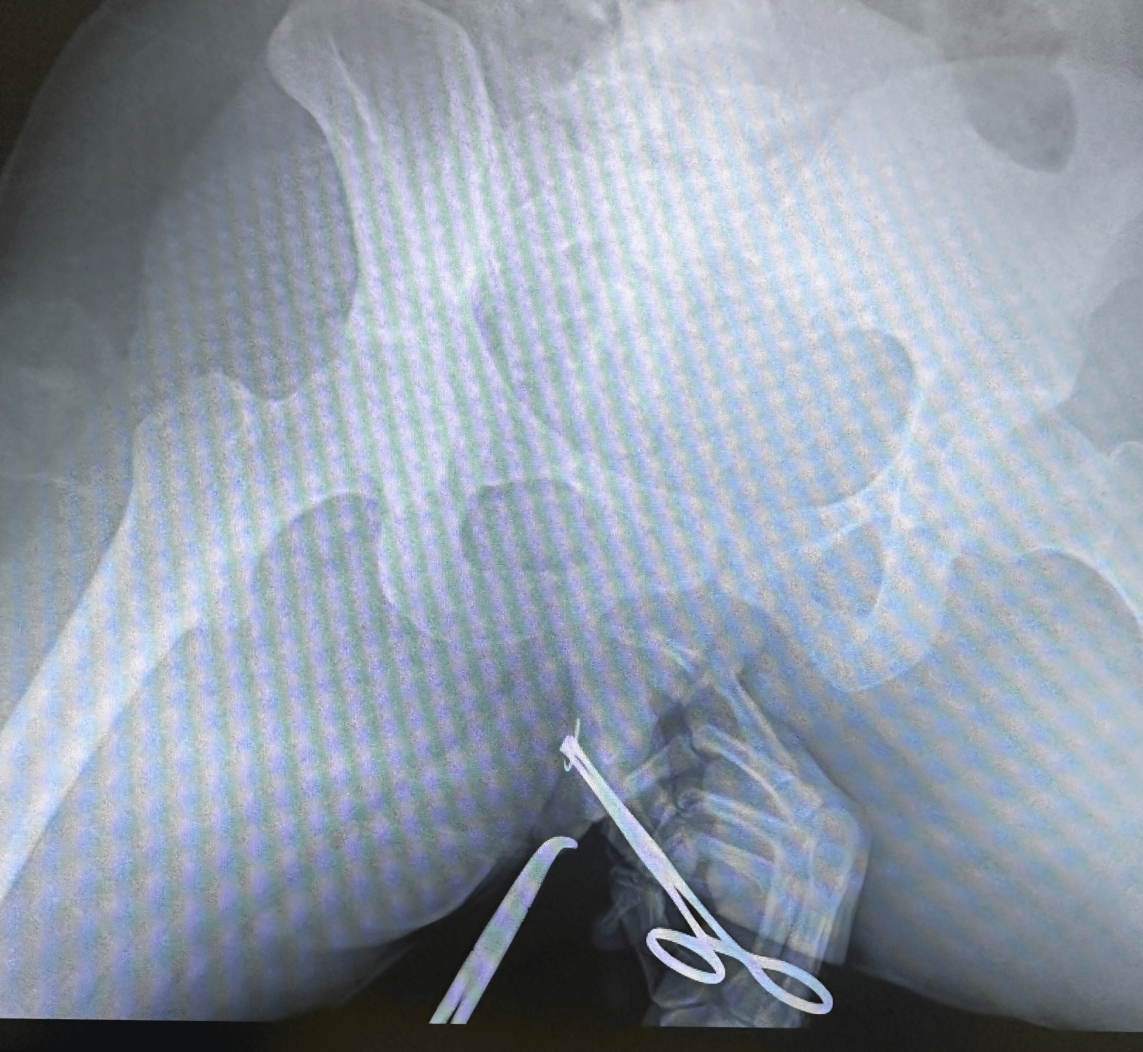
Radiographic-guided dissection of the needle fragment. Under real-time portable radiographic guidance, dissection was carefully performed toward the confirmed target area to expose the retained needle fragment prior to removal.
